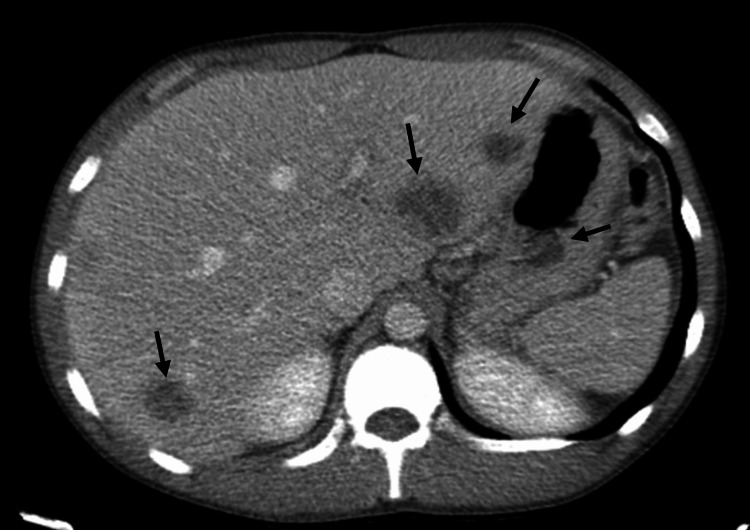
Multiple hypodense liver lesions on CT with contrast The patient’s initial CT on presentation to the ED revealed at least 10 bilobar hypodensities throughout the liver, raising concern for multifocal abscesses.
